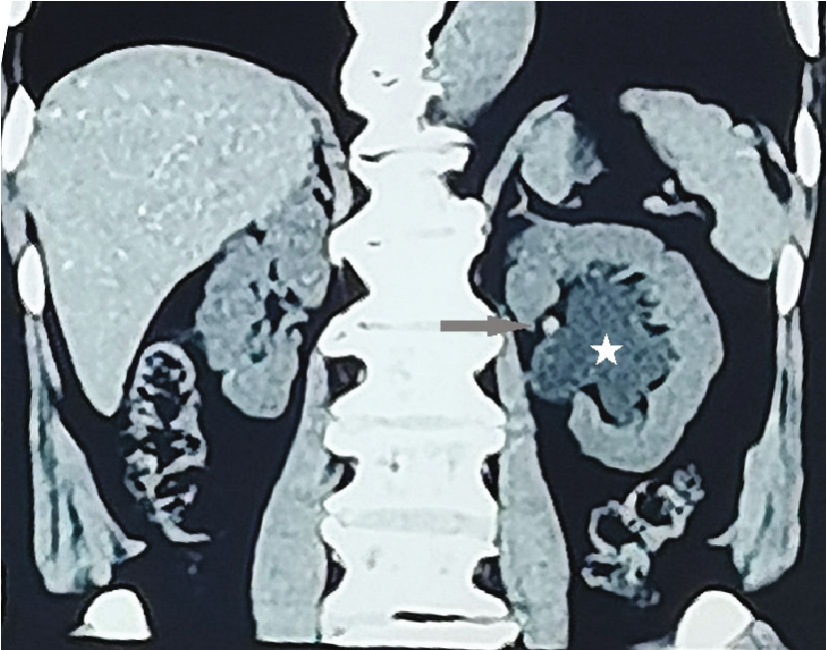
CT image in 2013 shows left renal pelvis effusion (white star) with a visible small stone shadow (gray arrow).
